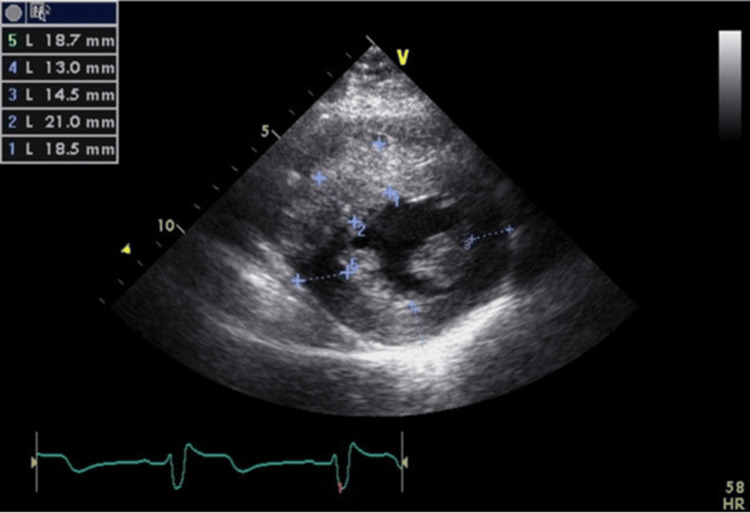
Echocardiography during the third pregnancy The maximum thickness of the left ventricular wall was 21 mm.
